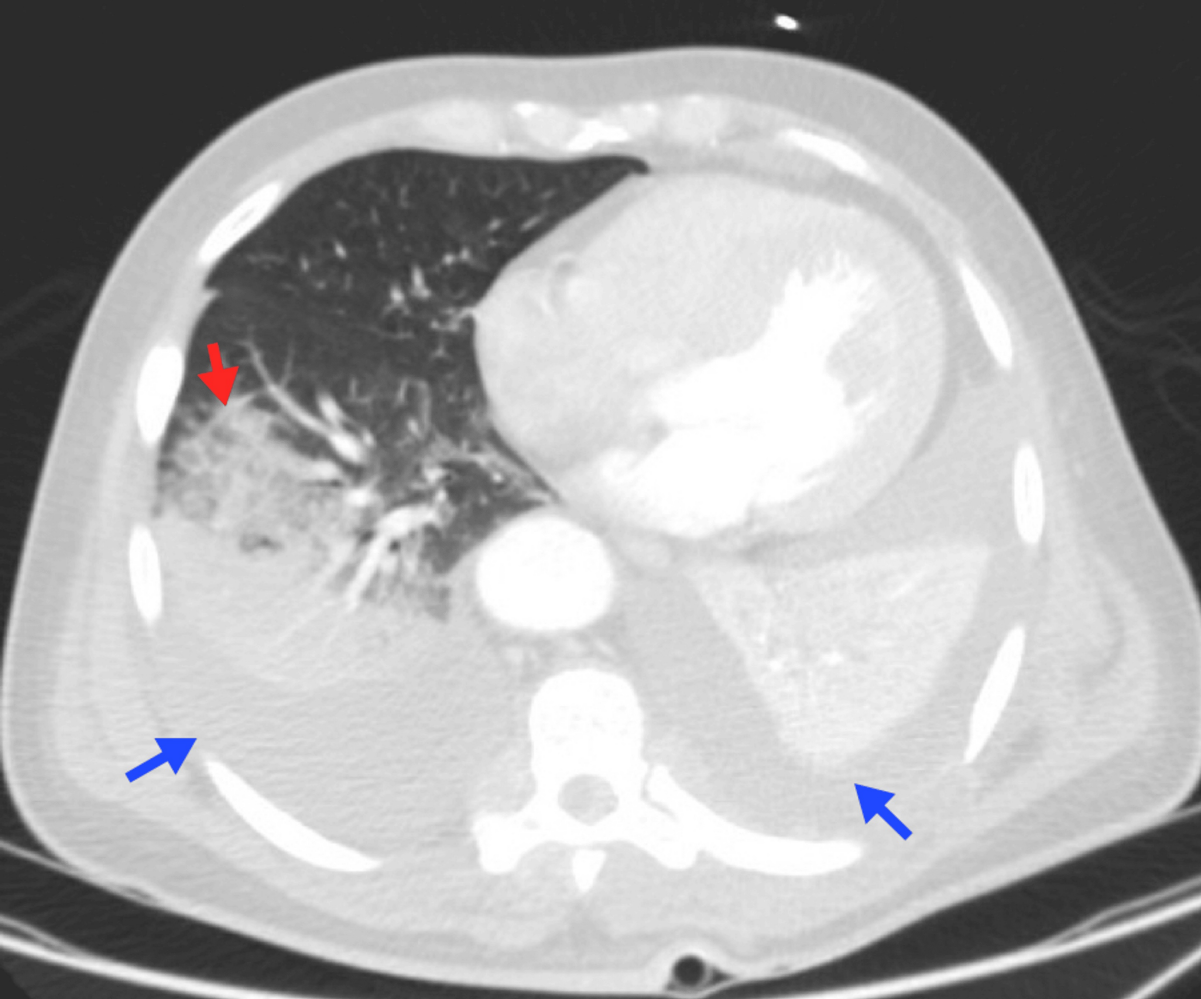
Bacterial pneumonia A 59-year-old male with lambda MM. Chest CT reveals extensive bilateral pleural effusion (blue arrows), along with alveolar consolidation in the inferior right lobe due to pneumonic involvement (red arrow). *Haemophilus influenzae* was isolated in microbiological studies. MM, multiple myeloma Image courtesy: Pulmonology Service of the Internal Medicine Department, University of Antioquia. Consent for open access publication was obtained from the patient.
